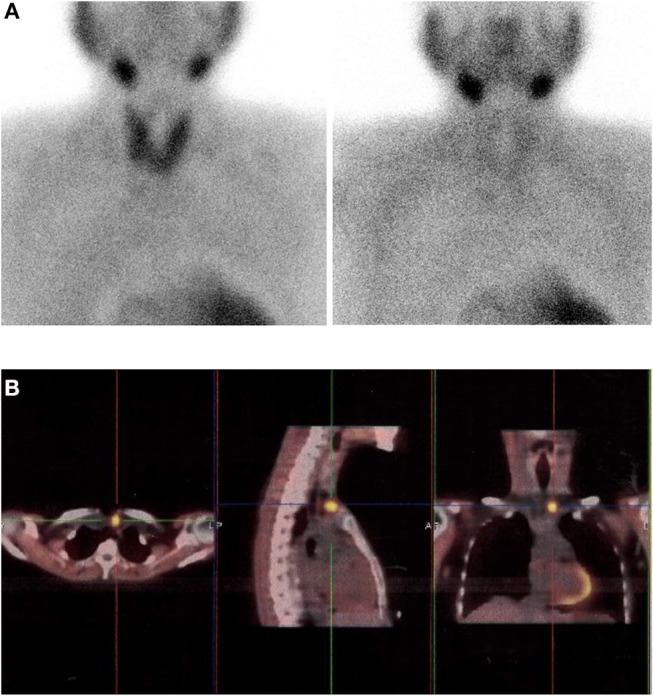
Images in a 63-year-old woman with primary hyperparathyroidism. (A) Dual-phase planar parathyroid images show diffuse sestamibi uptake in the thyroid gland, but no focal tracer uptake on early acquisition and uniform washout on delayed acquisition. (B) Axial, sagittal and coronal delayed-phase sestamibi SPECT/CT images show focal sestamibi uptake localizing to a single abnormal left upper parathyroid gland. The patient underwent minimally invasive parathyroidectomy for a single left upper parathyroid adenoma and experienced an appropriate drop in intraoperative parathyroid hormone.
